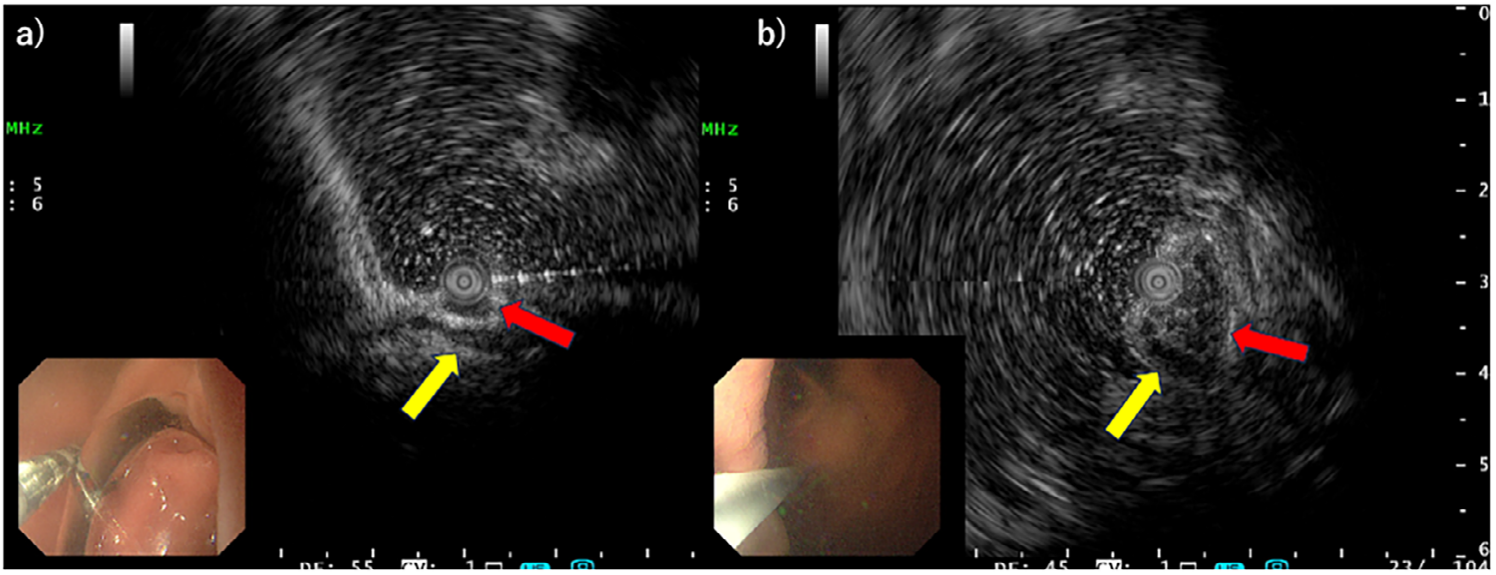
Endoscopic ultrasound images obtained using a 12‐MHz mini‐probe. The red arrow indicates the lesion. The yellow arrow indicates the fourth layer of the gastric wall. (a) Anterior wall of the antrum. (b) Cardia.
